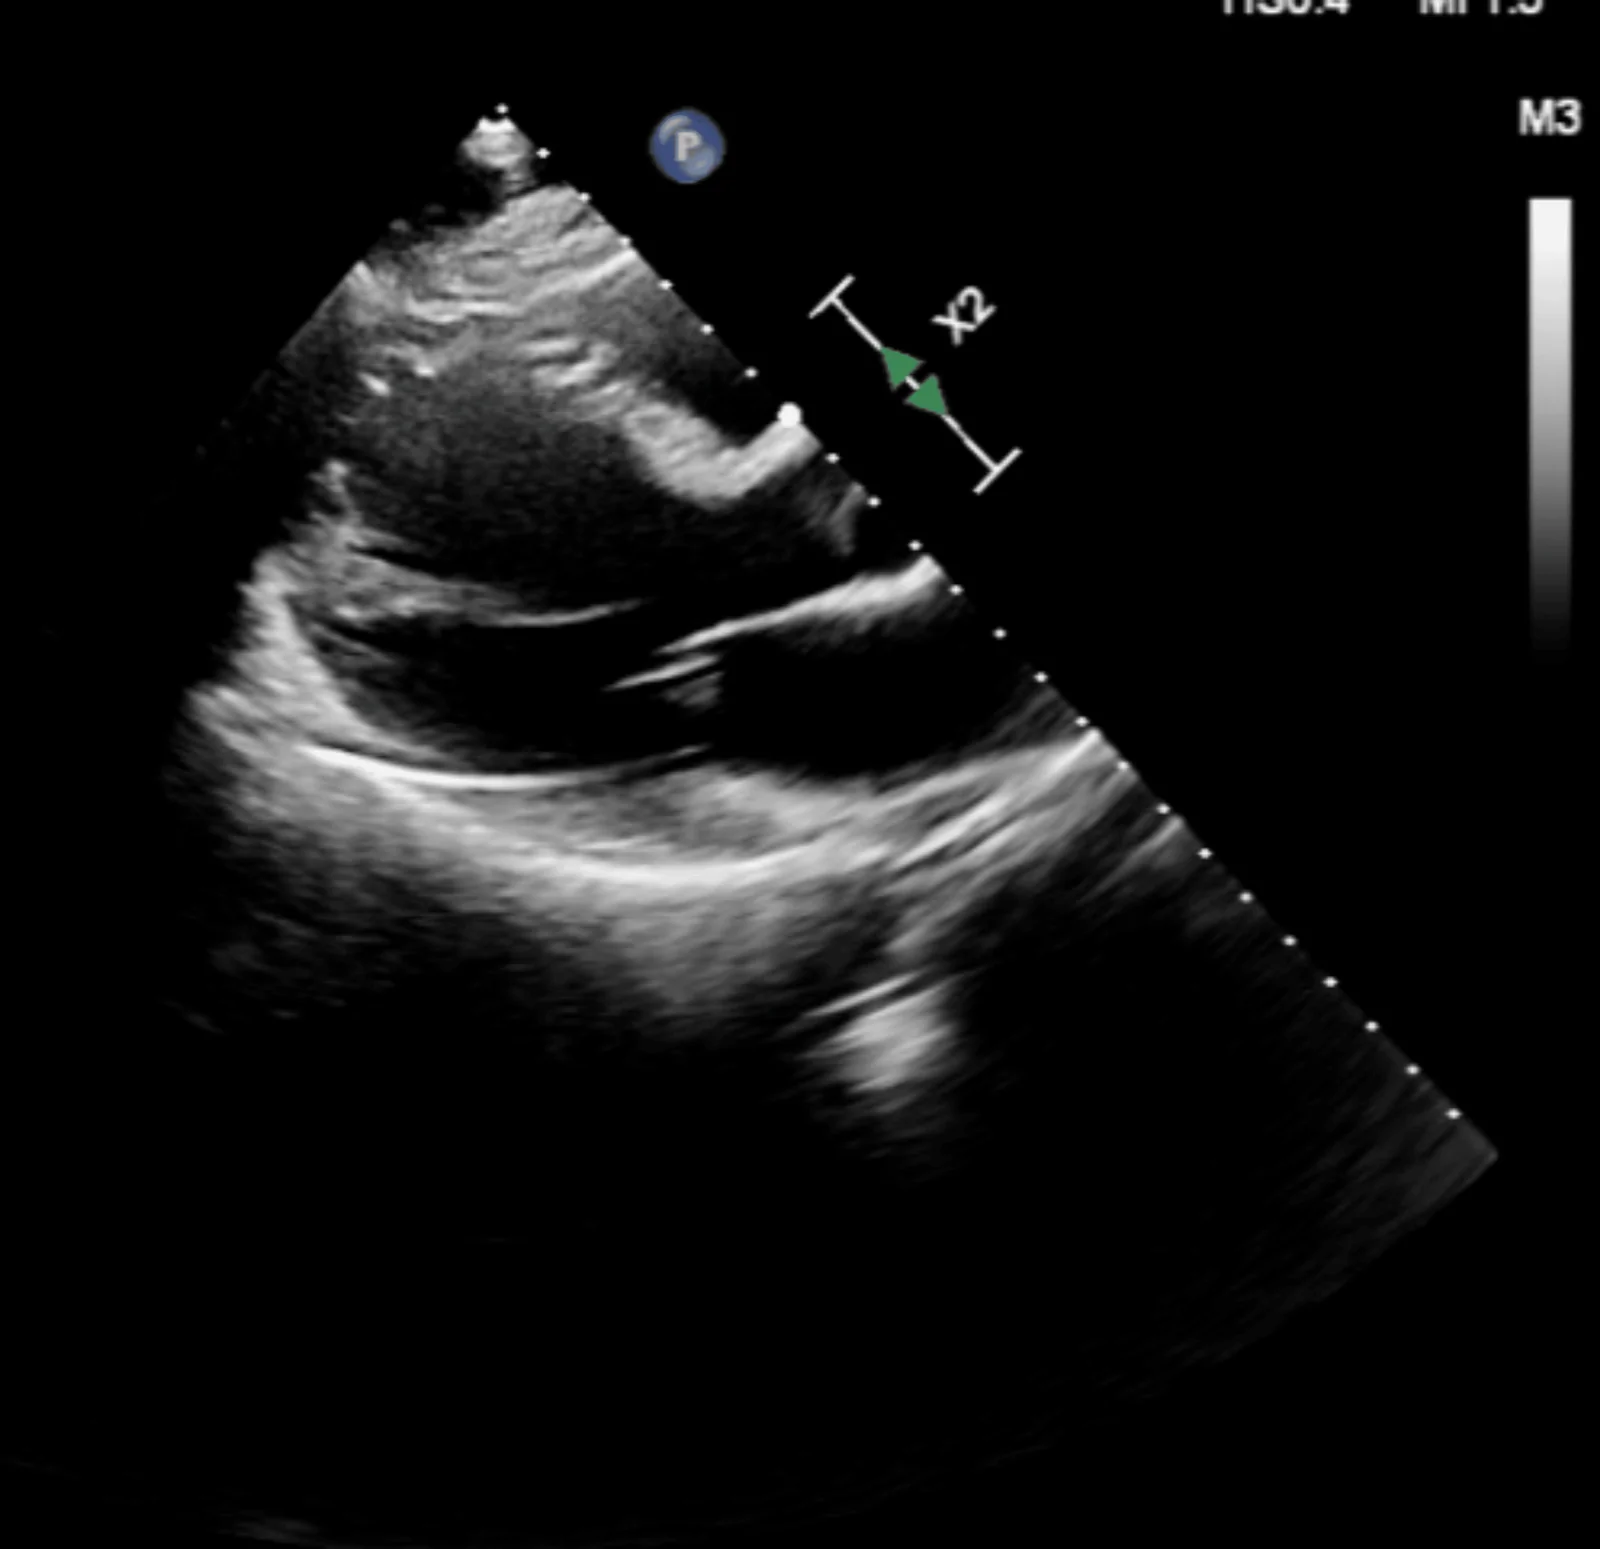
Postoperative transthoracic echocardiographic assessment following mitral valve repair
